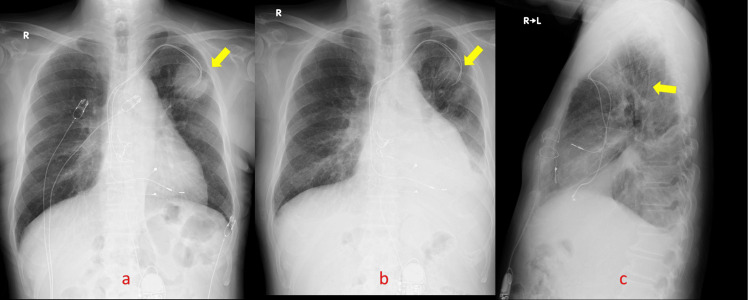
Chest X-ray films. a: The tumor (yellow arrow) three months after the end of radiation therapy (RT). b (frontal view) and c (lateral view): Arrows showing shrinkage of the tumor six months after RT.
